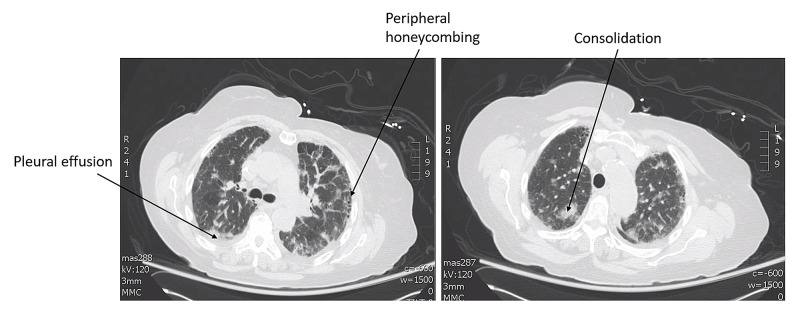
Computed tomography scan of the chest/abdomen/pelvis showed pulmonary fibrosis pattern, small bilateral perfusion, and multifocal areas of consolidation
